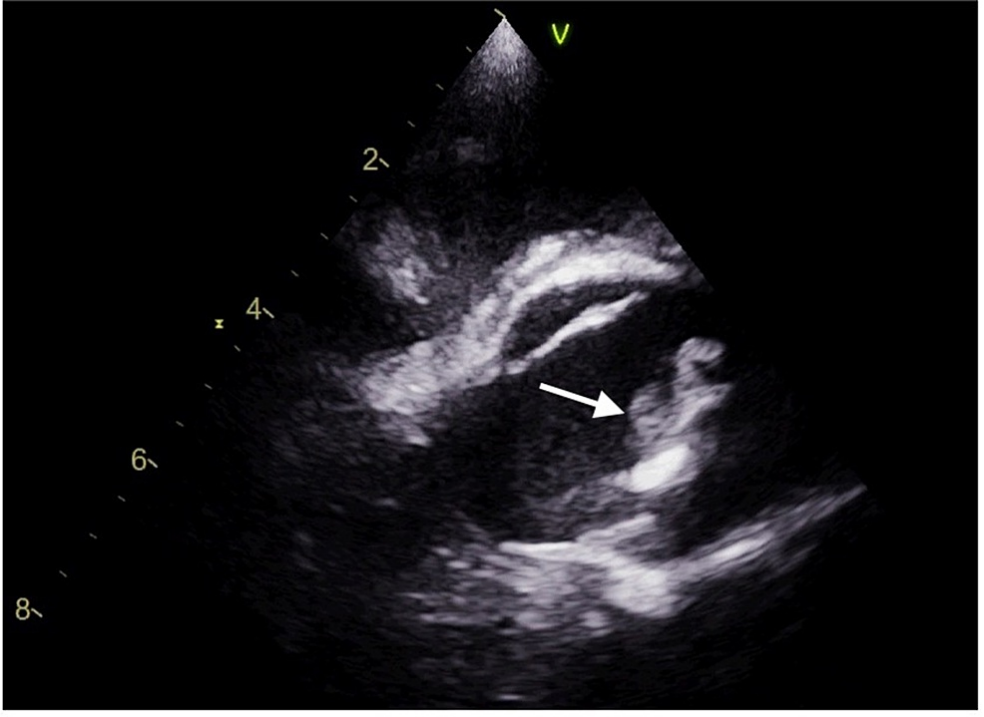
Parasternal long-axis view on transesophageal echocardiography revealing a large aortic valve vegetation (1.5 × 1.4 cm) and paravalvular abscess involving the aortic root
